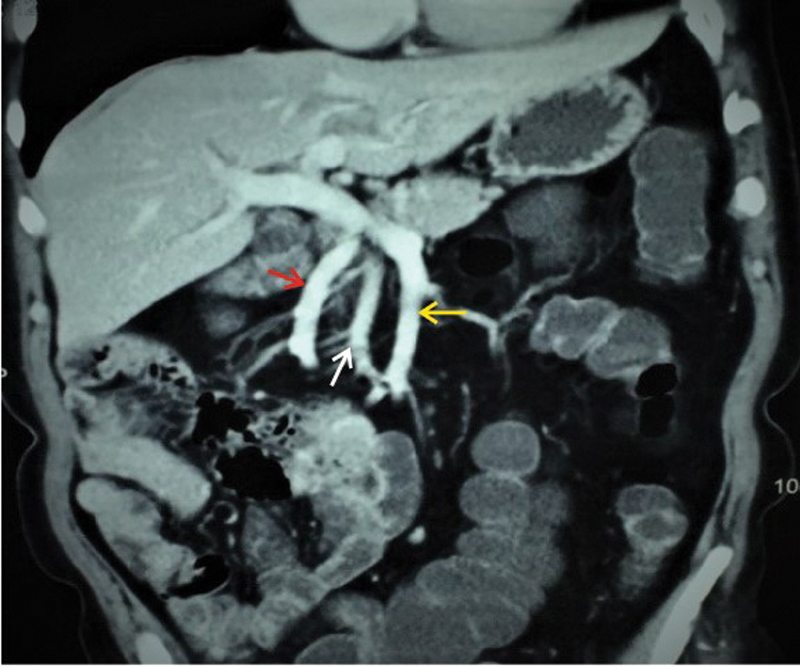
Coronal contrast-enhanced computed tomography scan portal venous phase image showing the ileal (yellow arrow) and jejunal branch (red arrow) of superior mesenteric vein and superior mesenteric artery (white arrow) behind and to the right of superior mesenteric vein.
